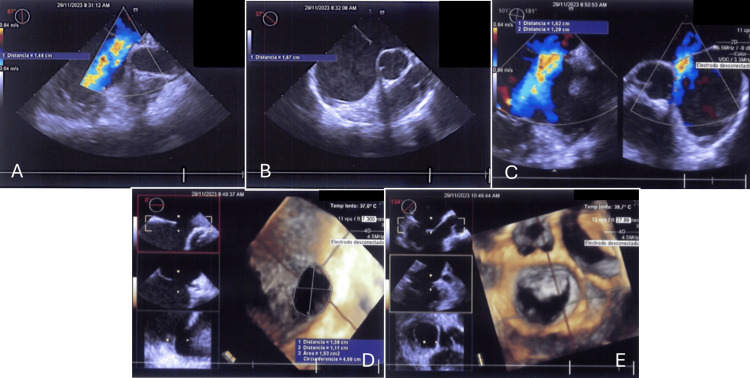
Preoperative transthoracic echocardiogram A-C: Ostium secundum-type atrial septal defect, with shunts from left to right, with agenesis of the inferior vena cava. D-E: Inter-atrial septum with a defect of 1.5 cm x 1.2 cm, with shunt from left to right, with a posterior edge of 1.6 cm, an upper edge of 0.6 cm, an inferior edge of 1.1 cm, and a short aortic edge of 4 mm.
